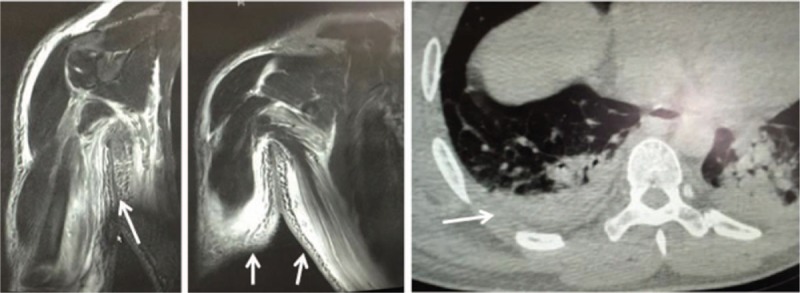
Magnetic resonance imaging (MRI) and computerized tomography (CT) displayed obvious soft tissue swelling of right forearm and thoracic walls (arrow).
